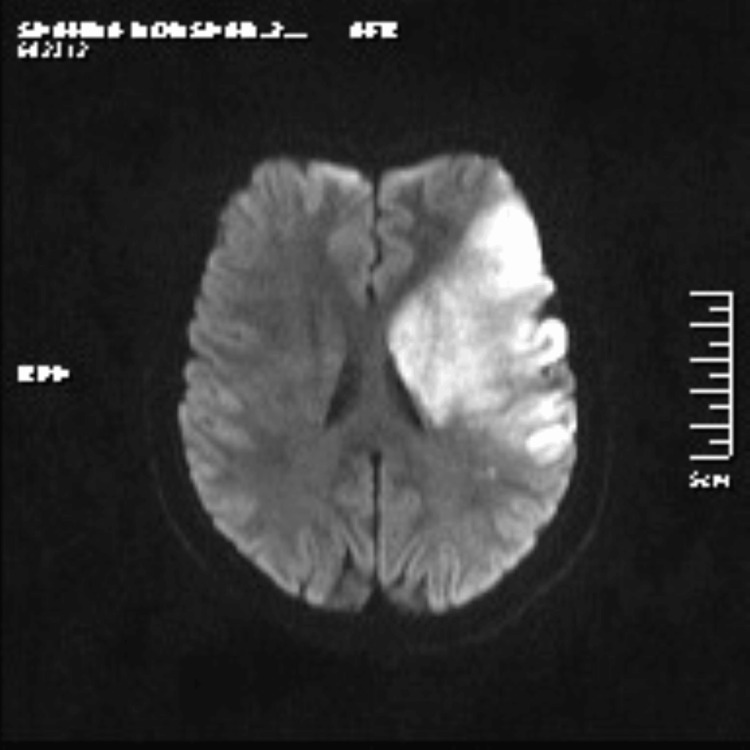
MRI brain showing acute infarct in the left fronto-parieto-temporal lobe and left capsulo-ganglionic region Moderate-sized acute infarct in the left middle cerebral artery territory involving left fronto-parieto-temporal lobe and left capsulo-ganglionic region. Mild mass effect is seen in the form of effacement of cortical sulcal spaces and mild compression of left lateral ventricle.
